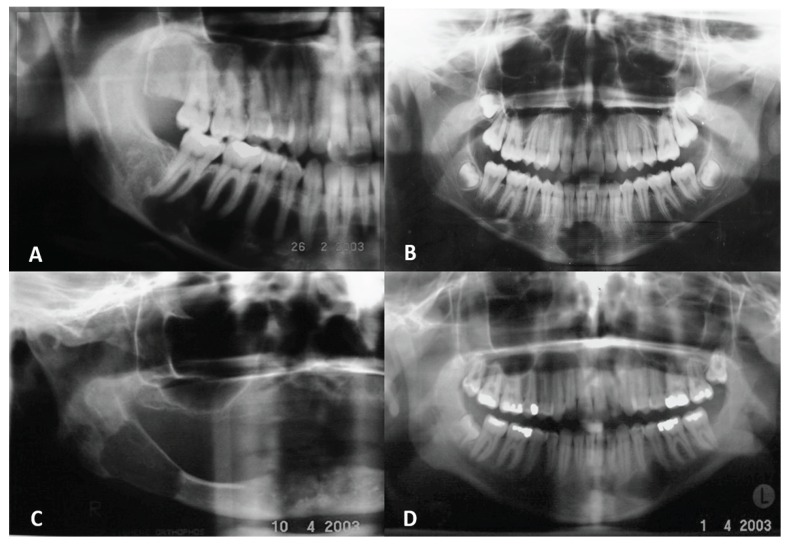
Panoramic radiographies of SBC and ABC. A. A classical aspect of SBC as a radiolucent
